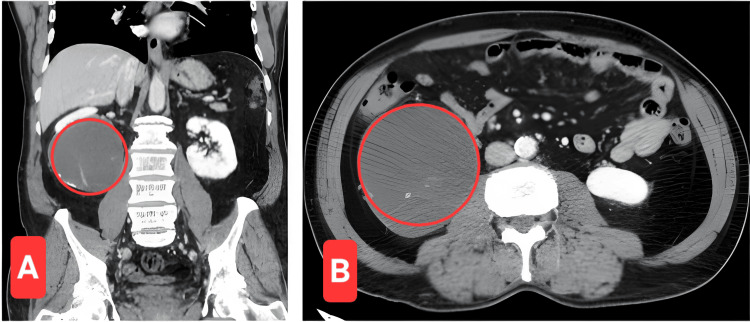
Contrast-enhanced abdominal CT shows a renal lesion circled in red in both coronal (A) and axial plane (B)
